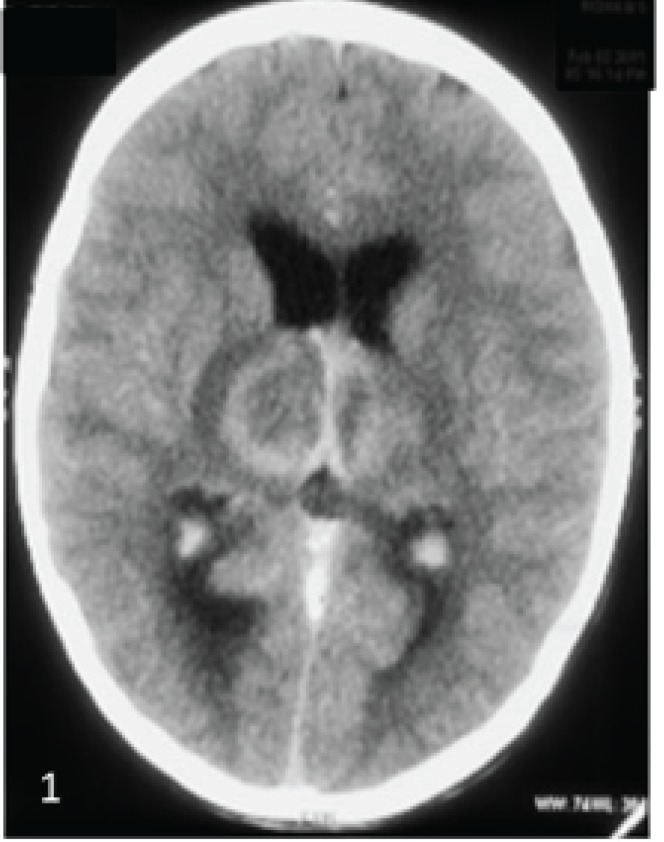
Axial non contrast CT Shows hemorrhagic infarcts with perilesional edema in bilateral thalami. Note the thrombosed hyperdense internal cerebral veins bilaterally & the thrombosed hyperdense straight sinus. Mild dilatation of lateral ventricle is noted
